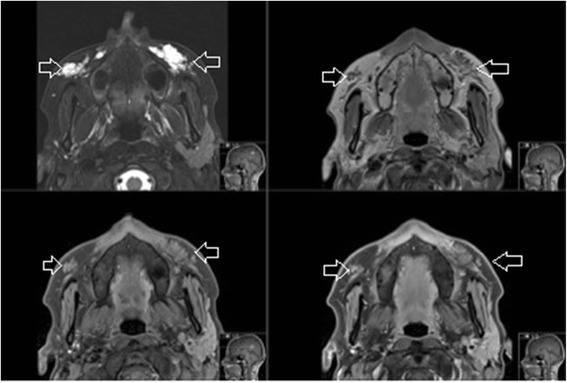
Collagen dermal filler in both infraorbital triangles (*open arrows*) on axial MRI (T2FS, T1, T1FS, T1FS with gadolinium)
